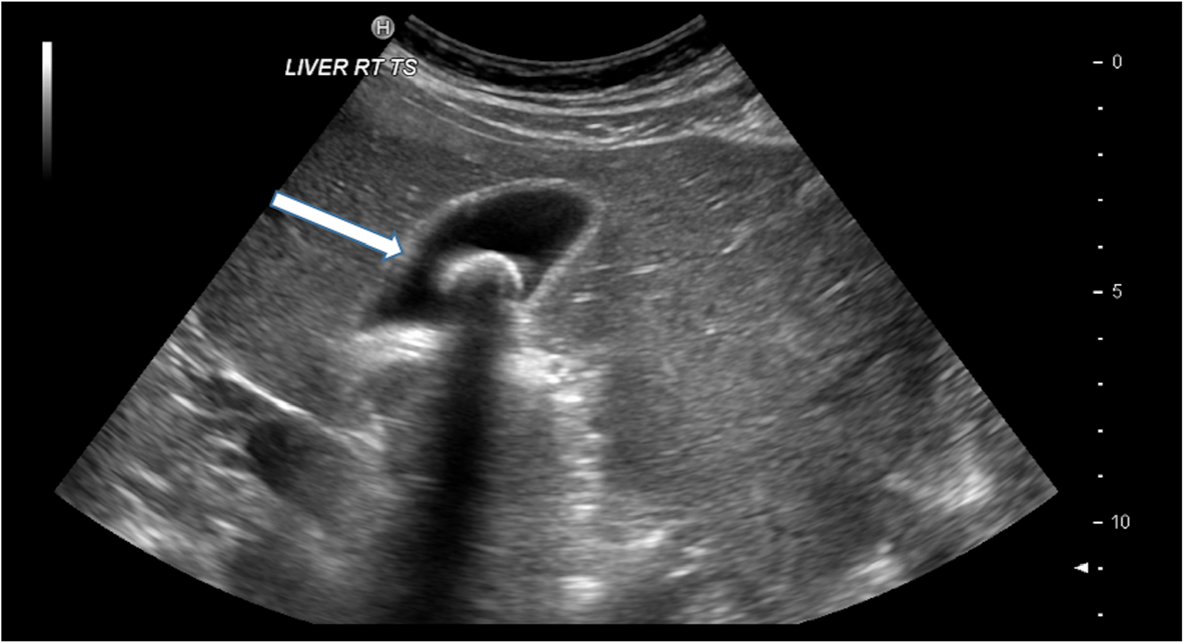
Sagittal ultrasound image of the gallbladder which demonstrates the typical appearance of a gallstone that is hyperechoic with posterior acoustic shadowing (arrow)
